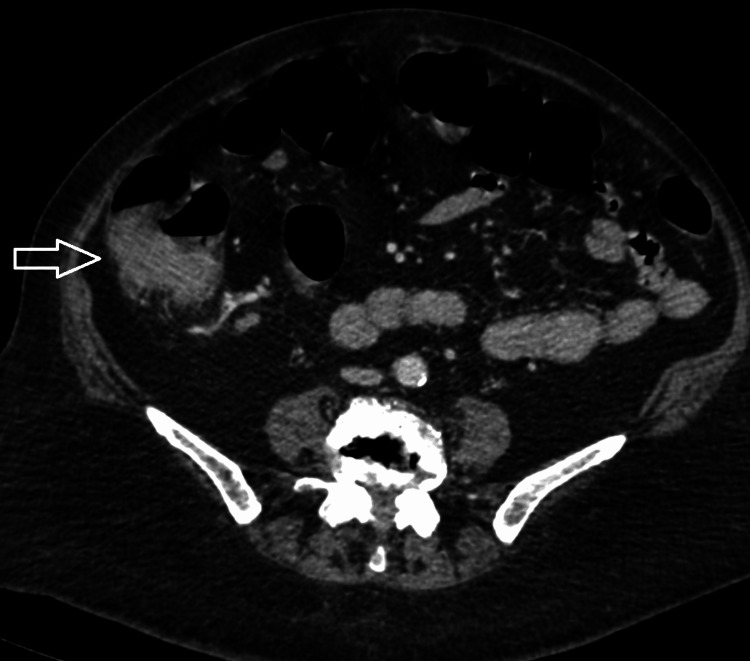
Axial view of caecal mass on computed tomography
